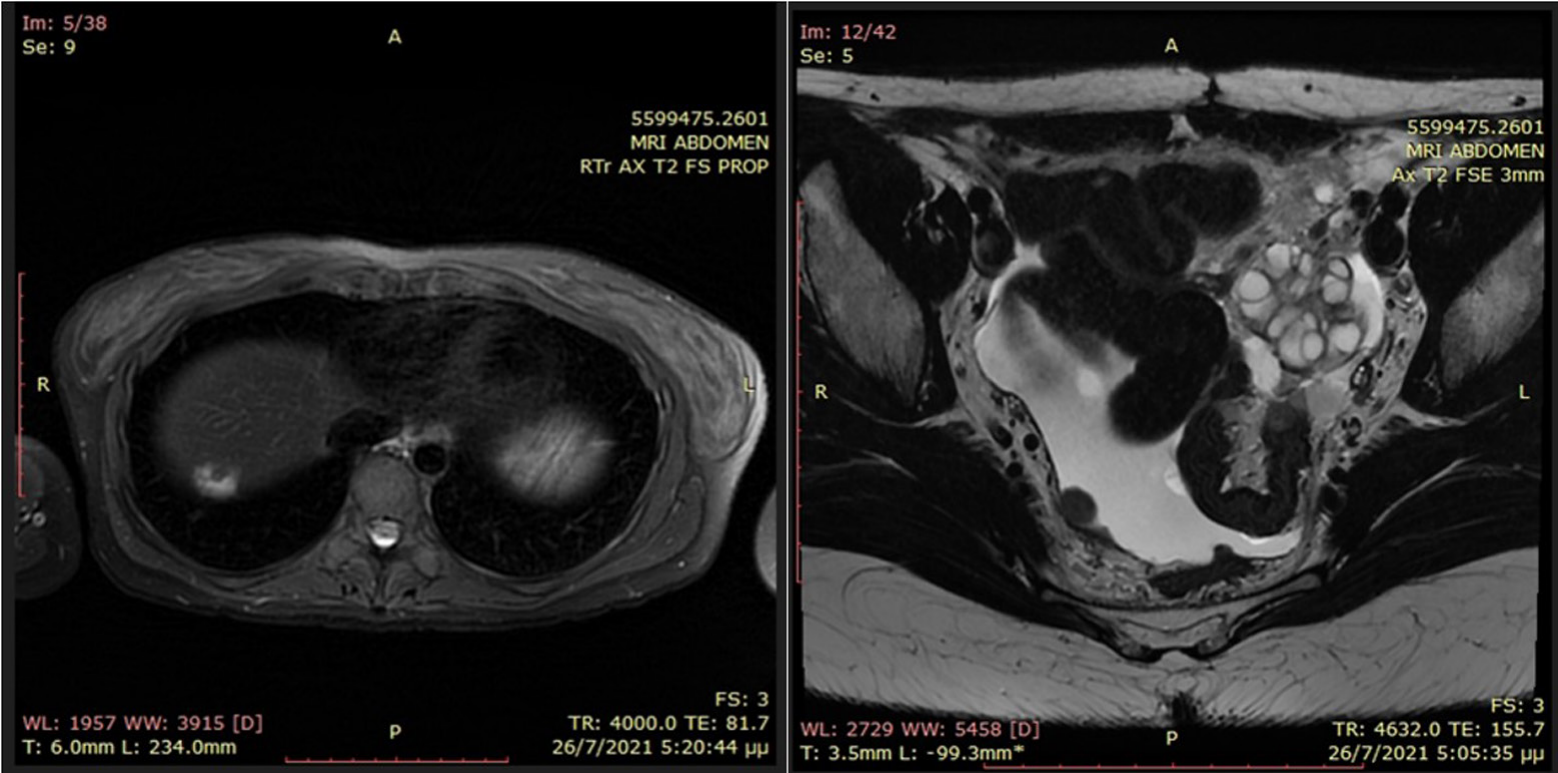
Abdomen MRI T2. (A) Lesion at the capsule of the liver (1.5 cm), below the diaphragm. (B) Mass complex at the left ovary and salpinx area (6.0 × 4.2 × 5.7 cm), with multiple cystic spaces (case 2).
